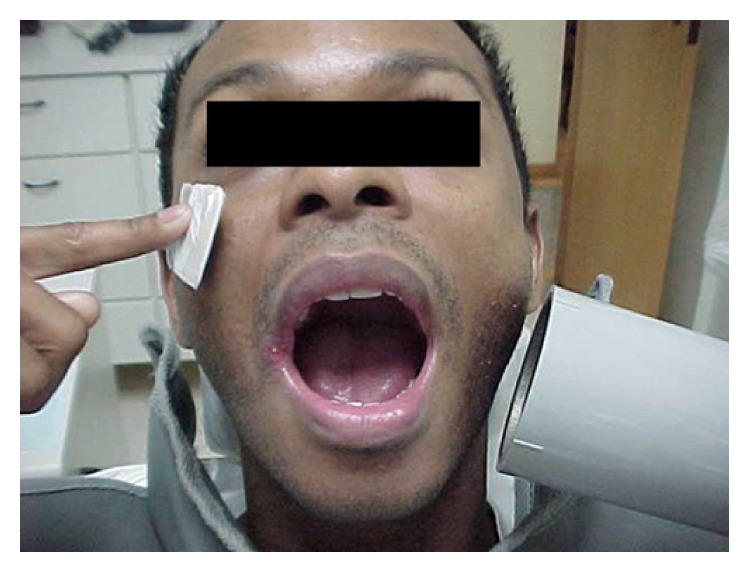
Extroral technique for final radiograph in root canal therapy of the maxillary molar.
